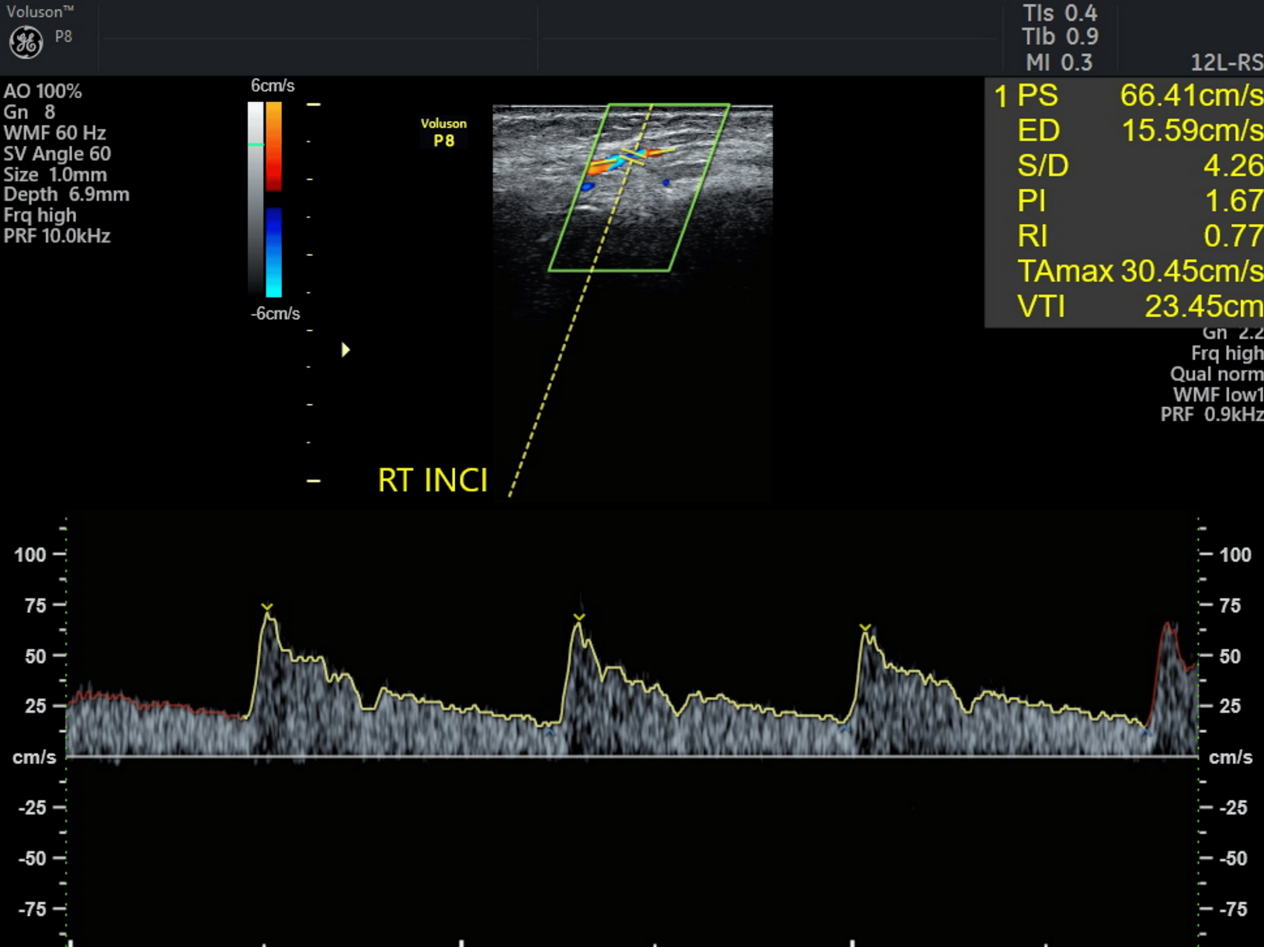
Ultrasound Doppler flowmetry of CAF + Mucograft on the third day. CAF: coronally advanced flap, CTG: connective tissue graft, PS: peak systolic velocity, ED: end-diastolic velocity, PI: pulsatility index, RI: resistive index, VTI: velocity time integral, RT INCI: right incisor.
